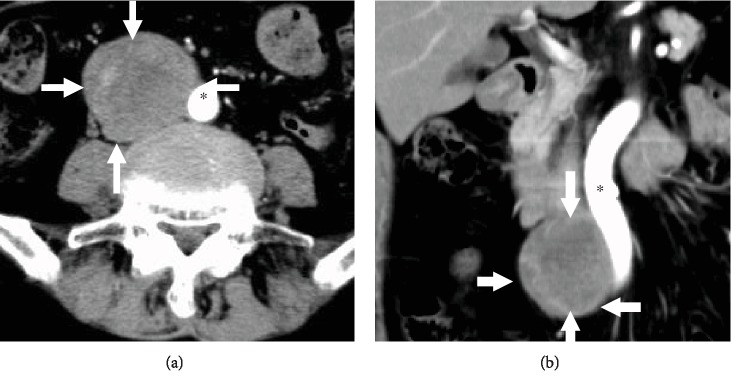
Computed tomography (CT) images at the recurrence revealed a large tumor (arrows) in the retroperitoneum, which was crossed to the aorta (^∗^). (a) Horizontal section and (b) coronal section.
